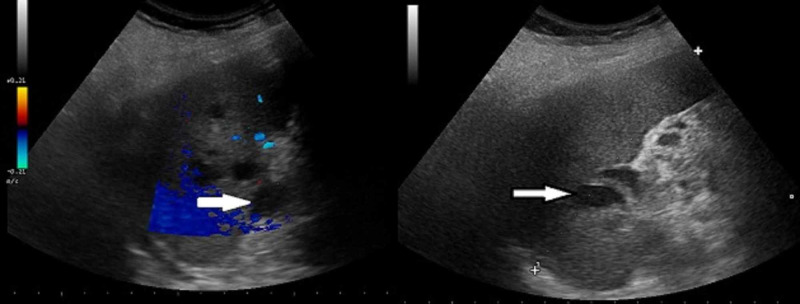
Ultrasonography showing multiple hypoechoic lesions in the spleen suggestive of splenic abscess (arrows).
